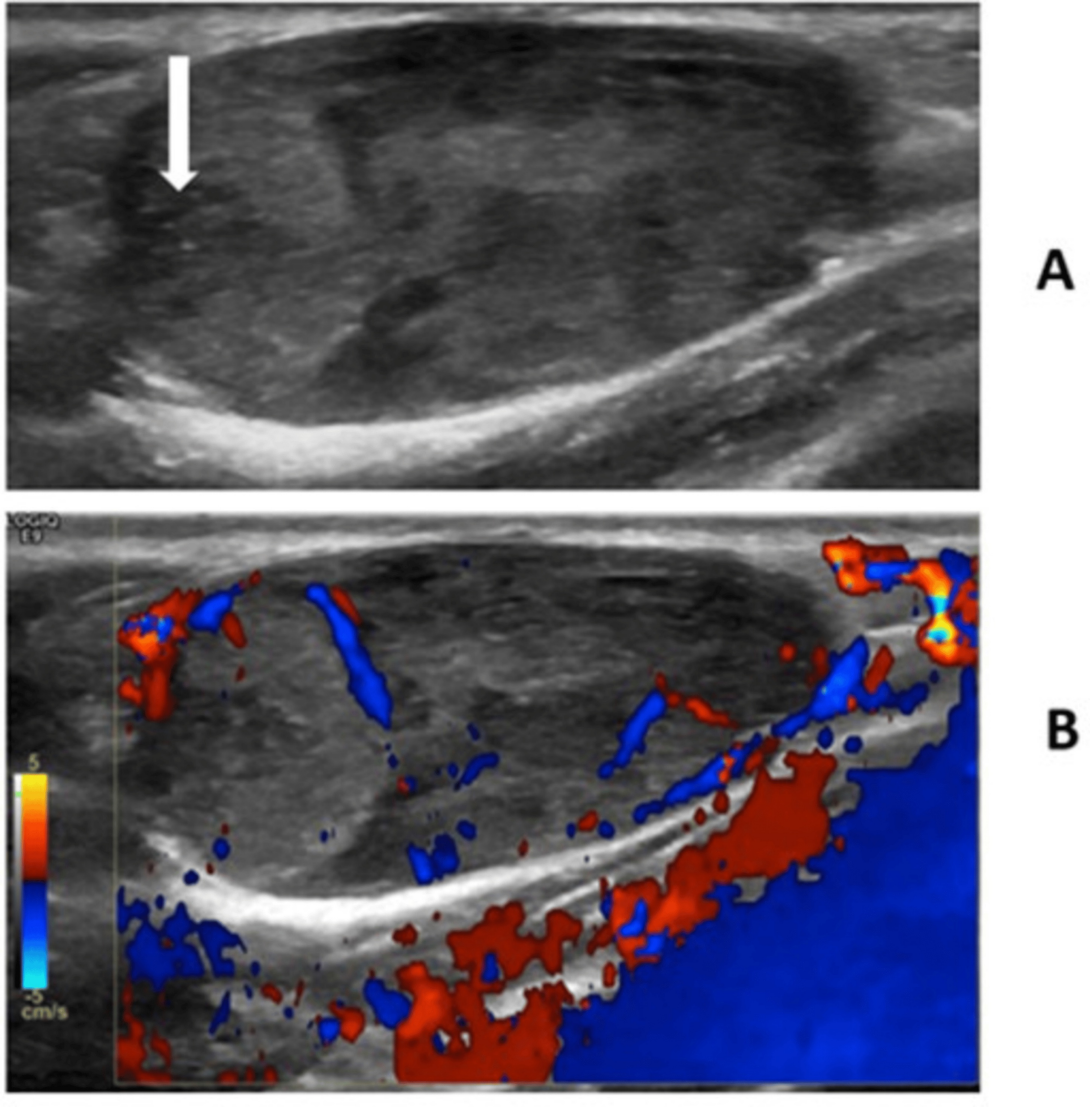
Grayscale (A) and Doppler (B) images of a lesion in the right upper back showing heterogenous echogenicities and regions of internal Doppler flow. There is a lack of diffuse increased vascularity typically seen in hemangiomas. There is questionable tiny calcification (arrow).
